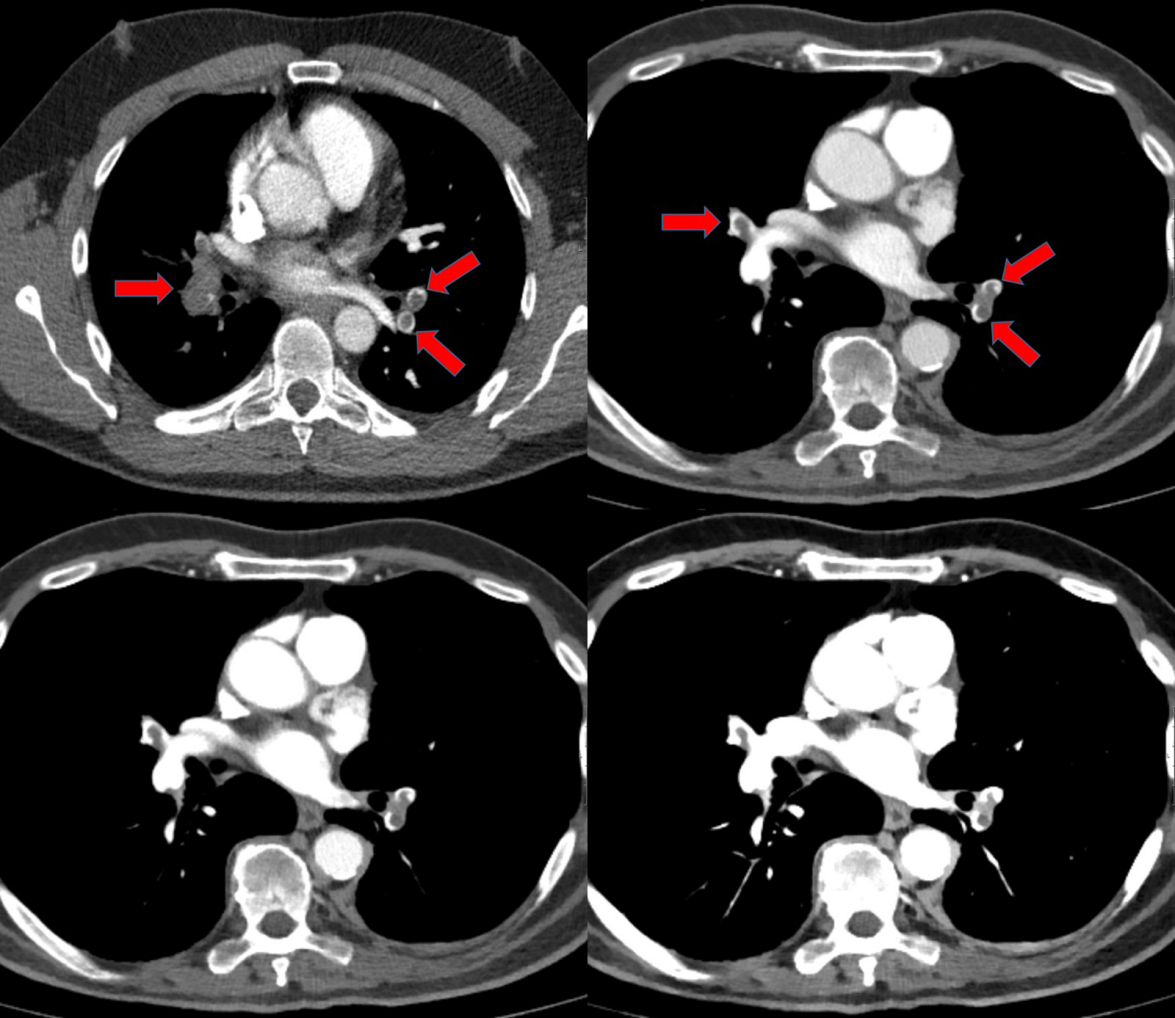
Comparison of two comparable patients scanned with the C-CT at 100 kVp (top left) and with the SD-CT at 120 kVp (top right and bottom). VMI of 70 keV (top right), 60 keV (bottom left) and 40 keV (bottom right) are shown. Pulmonary emboli are marked with red arrows. Note the clearly increasing contrast towards lower VMI-levels. As all images are shown at the same window setting (center: 100 HU, width 600 HU), VMI of 40 keV show a saturated iodine signal (For interpretation of the references to colour in this figure legend, the reader is referred to the web version of this article).
